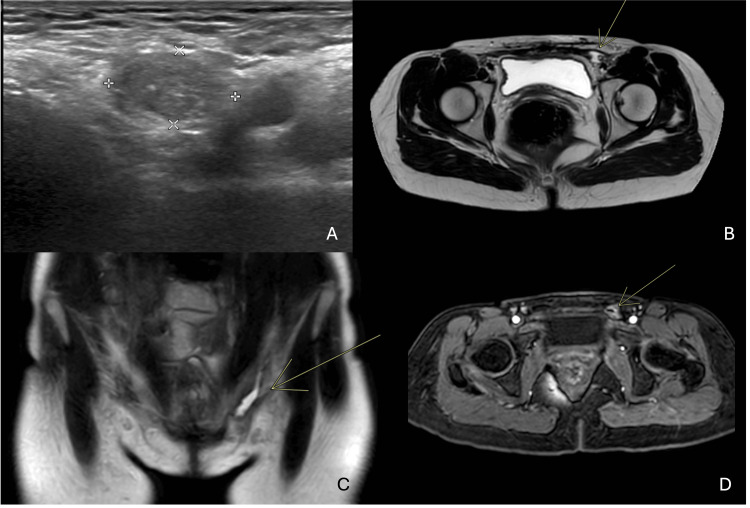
(A) A 1.8 × 1.1 cm oval, well-defined mildly hyperechoic structure is identified in the left inguinal region, located medial to the femoral artery with no internal vascularity. Axial (B) and coronal (C) T2-weighted images demonstrate a well-defined high T2 signal/cystic lesion in the left inguinal region extending toward the proximal portion of the canal of Nuck. (D) In the axial post-contrast T1-weighted image, there is mild peripheral wall thickening and enhancement suggesting a small canal of Nuck cyst with mild associated inflammation.
